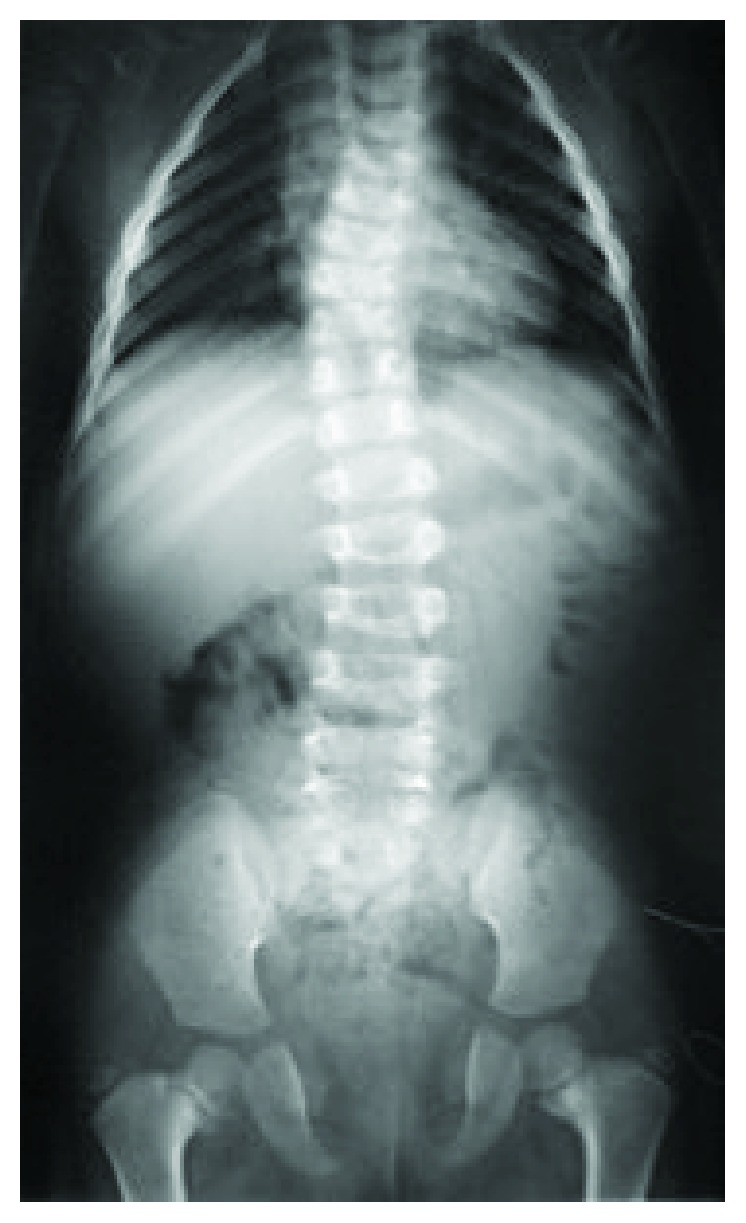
Widened pubic symphysis, bilateral coxa vara, and widened triradiate cartilage and sacroiliac joints can be seen in the pelvic radiographs (6.5 years).
